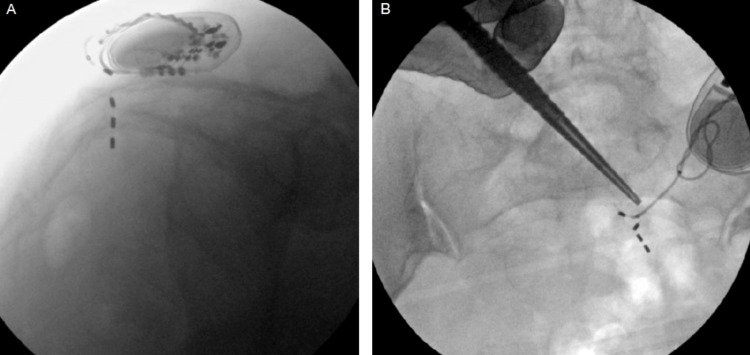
Scout pelvic radiographs prior to surgery A) Lateral view demonstrates worsened lead coiling and further posterior lead migration compared to pre-operative radiographs. B) AP view demonstrates lead fracture between electrodes two and three.
